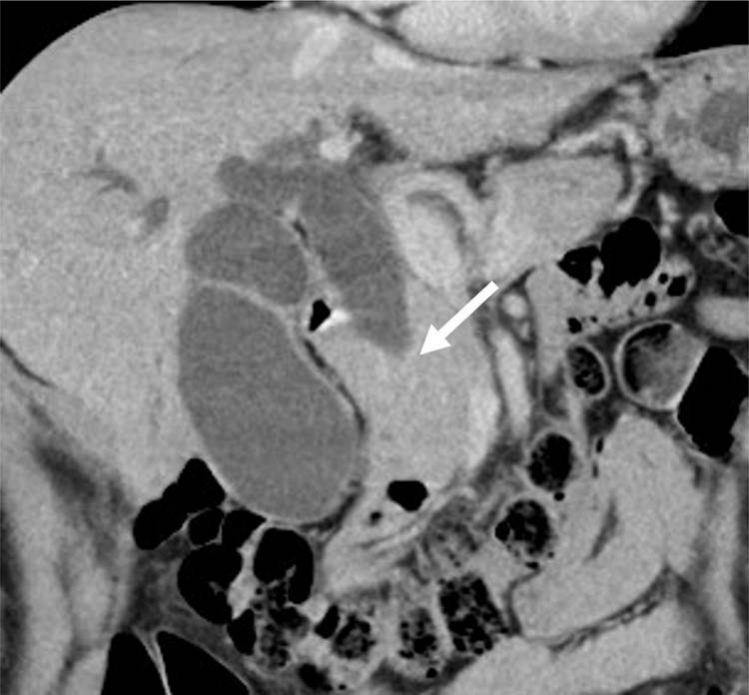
CT scan showed wall thickness and stenosis of the distal CBD (arrow).
